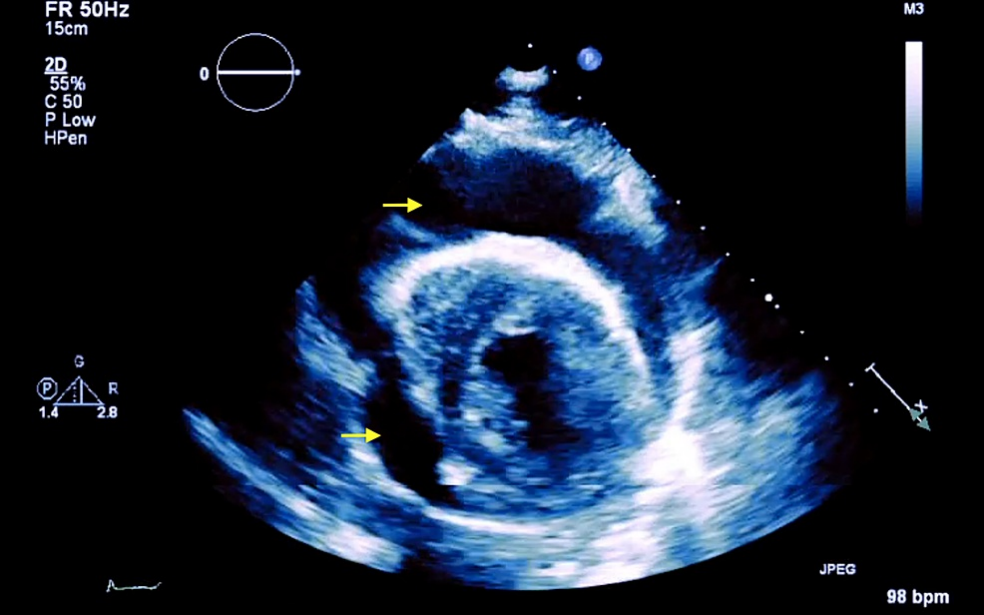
Transthoracic echocardiogram showing a large pericardial effusion (yellow arrows) in the setting of cardiac tamponade.
